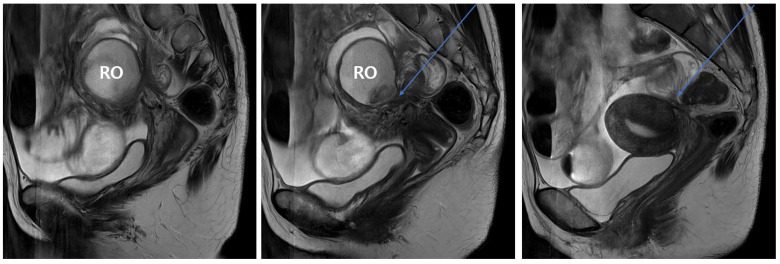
Serial sagittal pelvic MRI images demonstrating a 5.1 cm multiloculated cystic lesion in the right ovary (RO) with T1 hyperintensity and T2 shading, consistent with an endometrioma. Severe adhesions are observed among the right ovary, rectosigmoid colon, and posterior uterine wall. The right endometrioma shows dense adhesion to the distal ileum with a whirling appearance and a transition point (blue arrows), resulting in small bowel obstruction. Findings are consistent with deep-infiltrating endometriosis. Abbreviations: MRI, magnetic resonance imaging, RO, right ovary.
